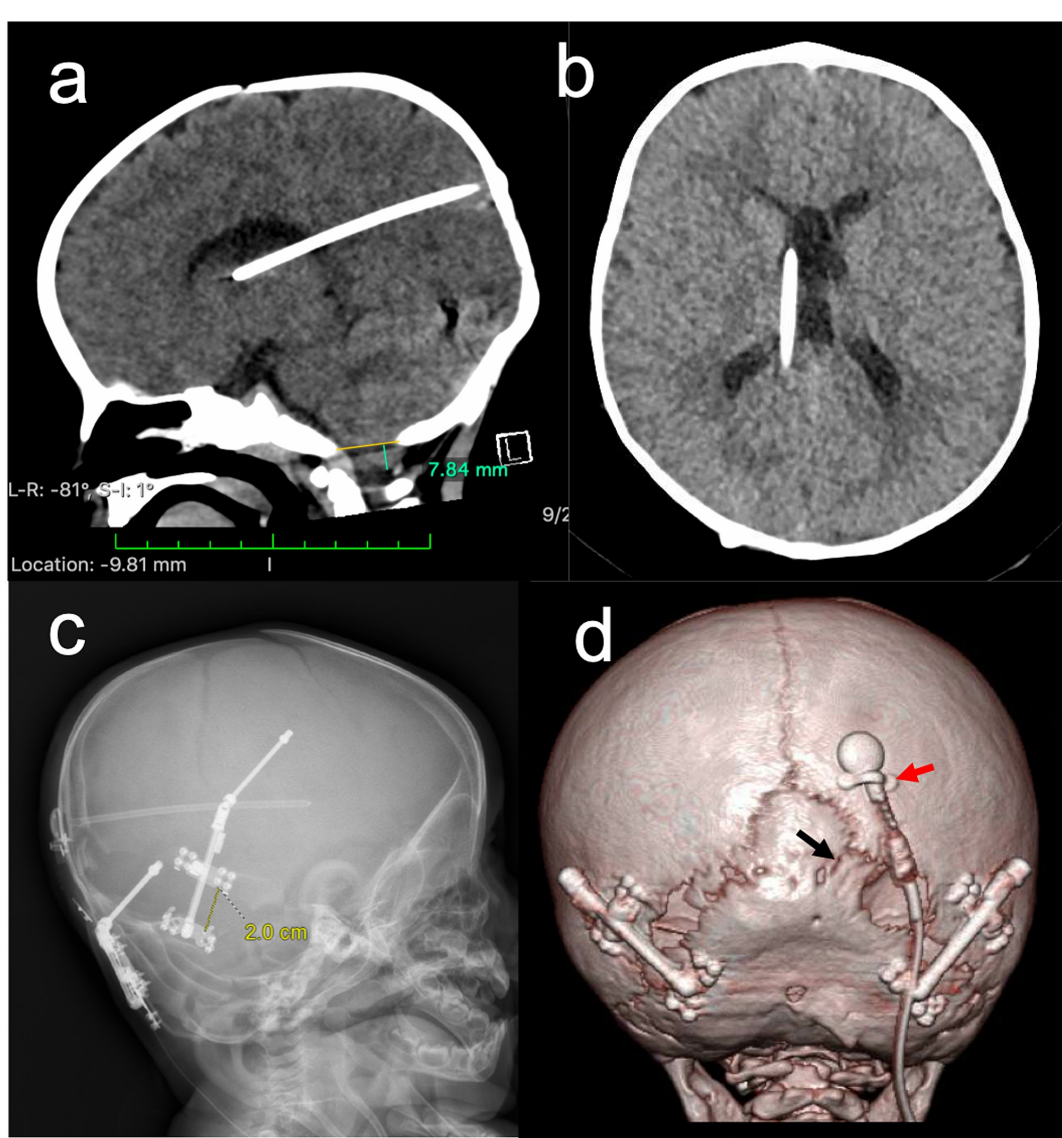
Postoperative CT and x-ray Axial CT image (a and b), x-ray (c) and 3D modeling (d). Panel (c) demonstrates bilateral distractor placement achieving 20 mm of distraction at two weeks' post-op. 3D reconstruction (d) of CT imaging collected at 2.5 months' post-op (a and b) shows evidence of bony bridging across the strip craniectomy (black arrow). Osteotomy was taken to the asterion bilaterally. Shunt is affixed to the skull using a wishbone cranial plate (red arrow). Shunt shows no evidence for migration with distraction.
